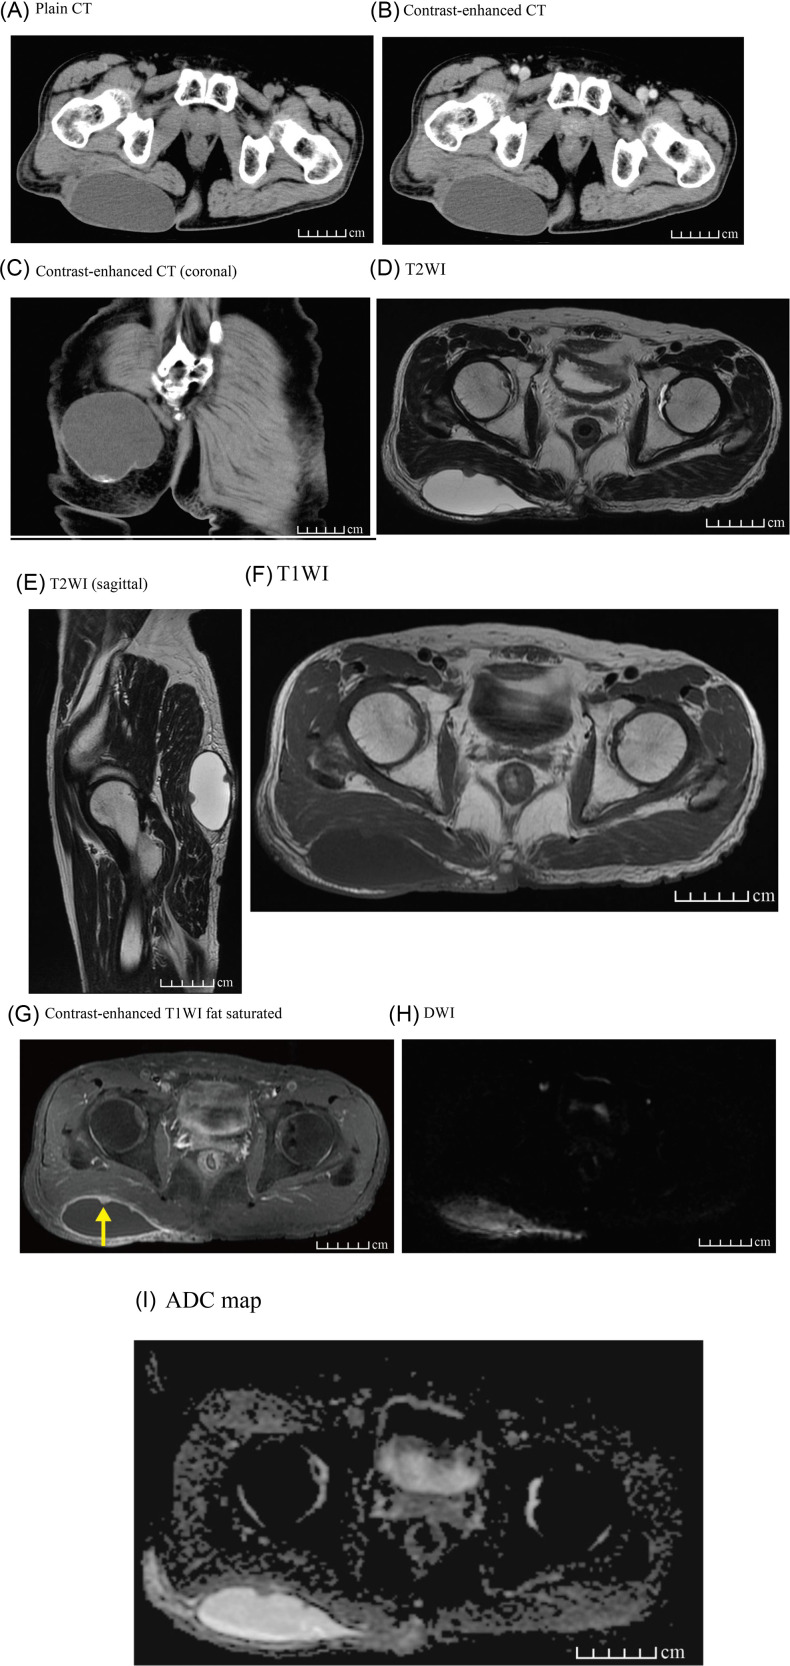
Case 2, a male in his 60s. Computed tomography images are shown in (A) plain CT, (B) contrast-enhanced CT, and (C) contrast-enhanced CT on the coronal plane. Magnetic resonance imaging (MRI) is presented in (D) T2-weighted image (T2WI), (E) T2WI on the sagittal plane, (F) T1-weighted image (T1WI), (G) contrast-enhanced T1WI fat-saturated (a nodule is indicated by yellow arrow), (H) diffusion-weighted image (DWI) and (I) apparent diffusion coefficient (ADC) map.
